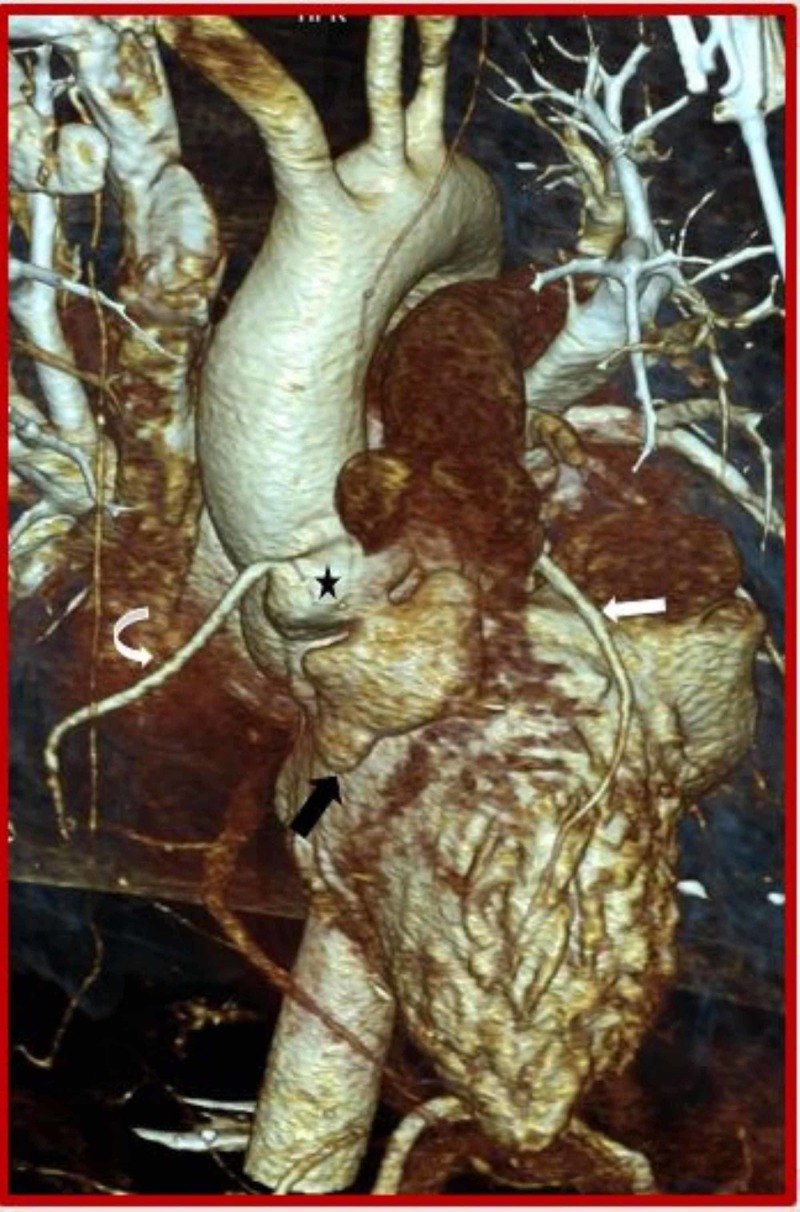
Cardiac CT (3D VRT) demonstrates ruptured right sinus of Valsalva aneurysm (asterisk) dissecting into the interventricular septum (black arrow). CT: Computed tomography; RV: Right ventricle; Curved arrow: Right coronary artery; Straight white arrow: Left coronary artery.
